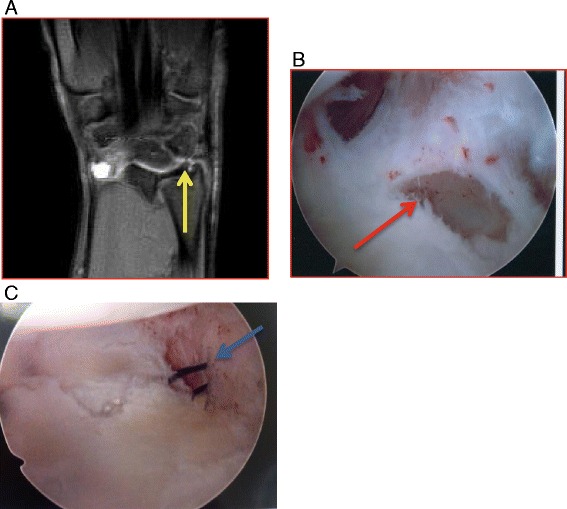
a A T2-weighted coronal sequence of a wrist MRI revealing an ulnar-sided peripheral tear of the TFCC (*yellow arrow*). b Arthroscopy view from the three to four portal showing the peripheral tear (*red arrow*). c Intraoperative arthrosopic image during an arthroscopic-assisted outside-in repair using PDS suture (*blue arrow*)
